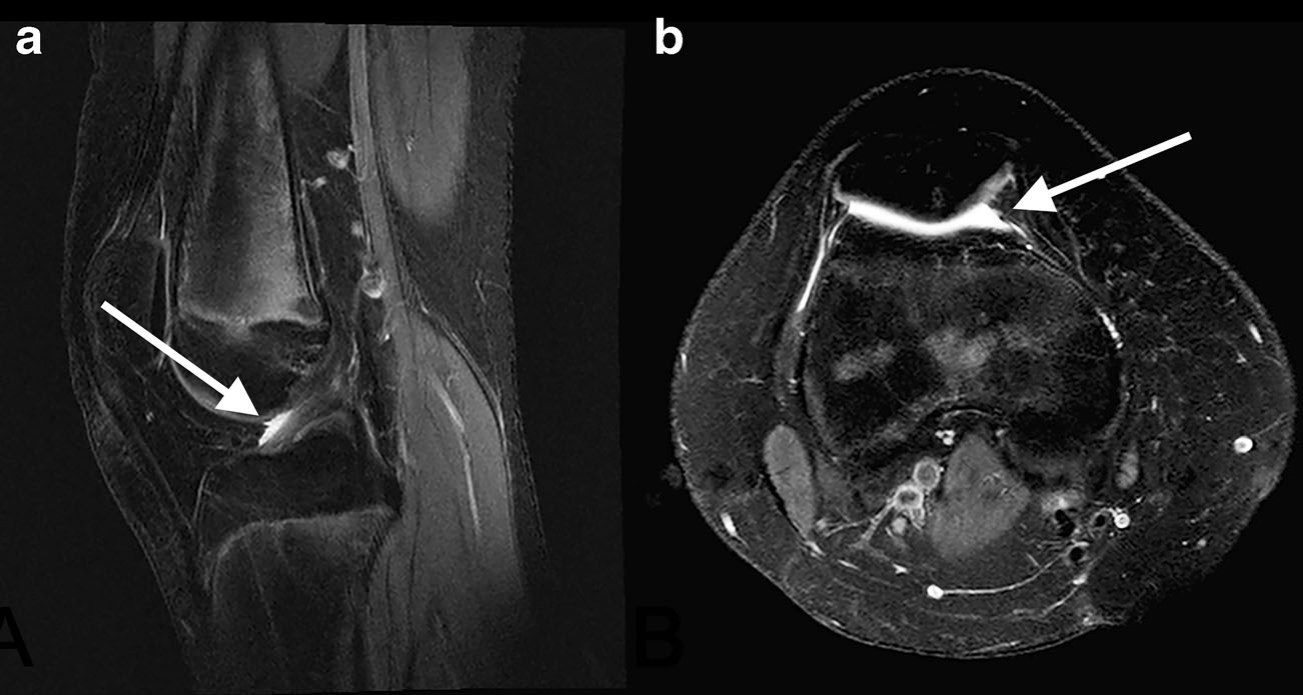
Joint fluid in two different healthy children (arrows). (A) Sagittal and (B) axial T2-weighted fat-saturated images
